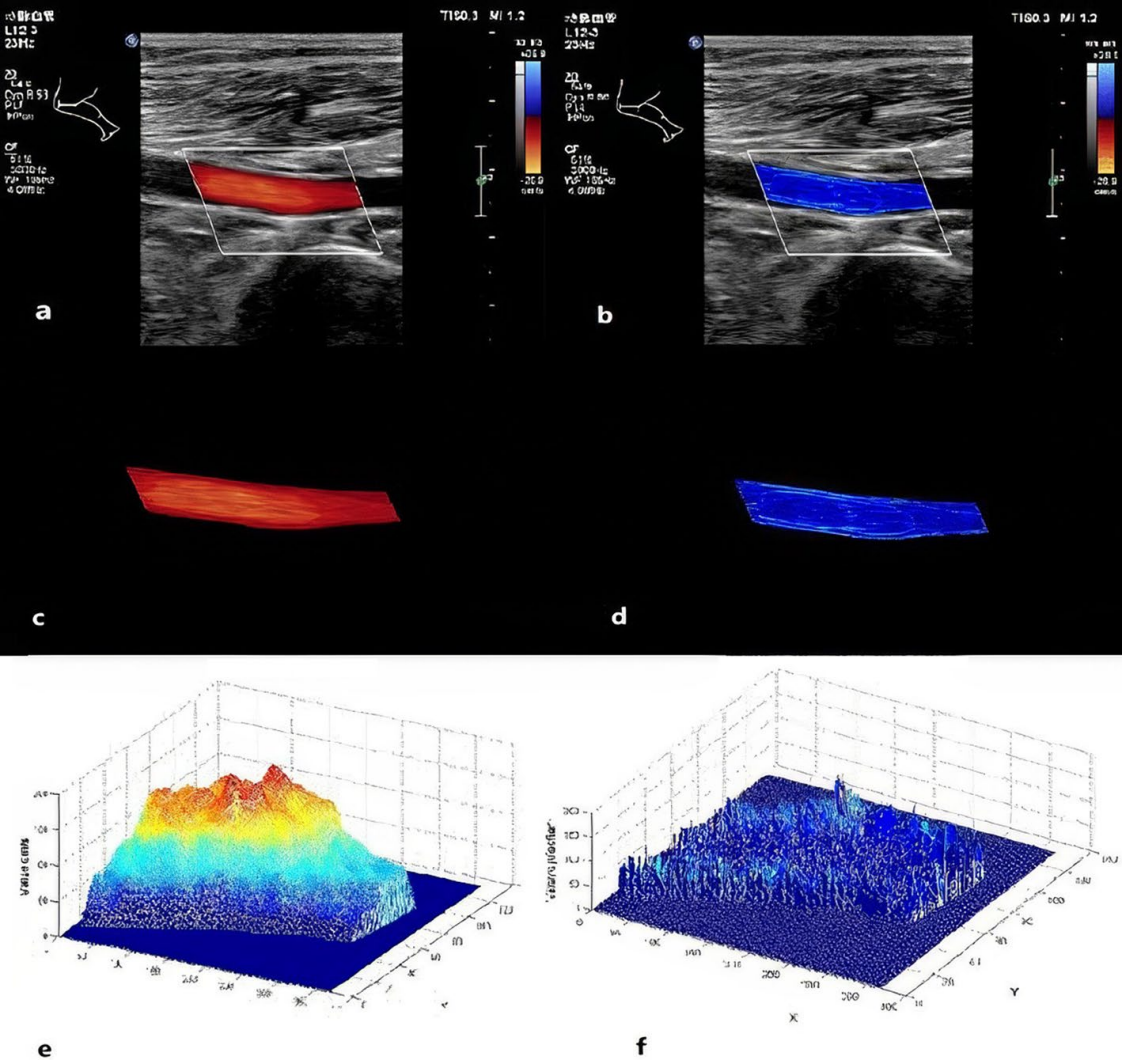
a Doppler flow image of the popliteal artery; b WSS distribution map fused with the Doppler flow image of the popliteal artery; c Segmentation map of the popliteal artery Doppler flow; d 2D distribution map of WSS in the popliteal artery; e 3D spatial map of Doppler blood flow velocity in the popliteal artery; f 3D spatial distribution map of WSS in the popliteal artery
